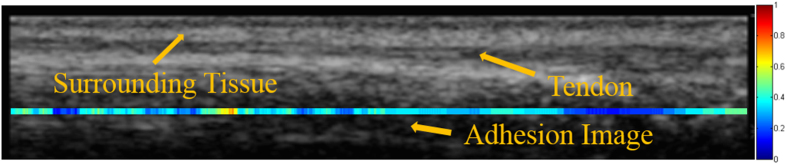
Ultrasound image of the EDC tendon and its surrounding tissue from a healthy individual. The surrounding tissue can be identified by its hyperechogenic structure while the tendon can be recognized by its less echogenic characteristics, fiber-like structure, and larger movement compared to other tissues in the region. The adhesion image indicates the locations of the tendon where the occurrence of adhesion is suspected; that is, where the relative displacement ratio is >0.6. A smaller ratio indicates a better gliding ability (relative displacement ratio of <0.6), and so the adhesion image for a healthy individual would generally appear green or blue.
